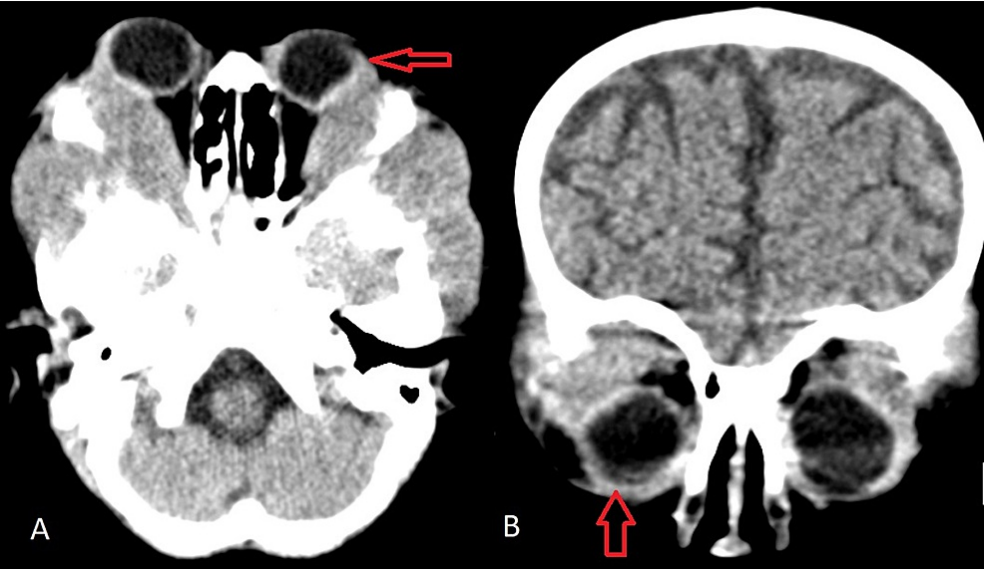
Brain and orbits CT -- (A) axial view with signs of orbital hyperdensity. (B) Coronal view showing bilateral heterogeneous hyperdense lesion in the lateral part of the orbits.
